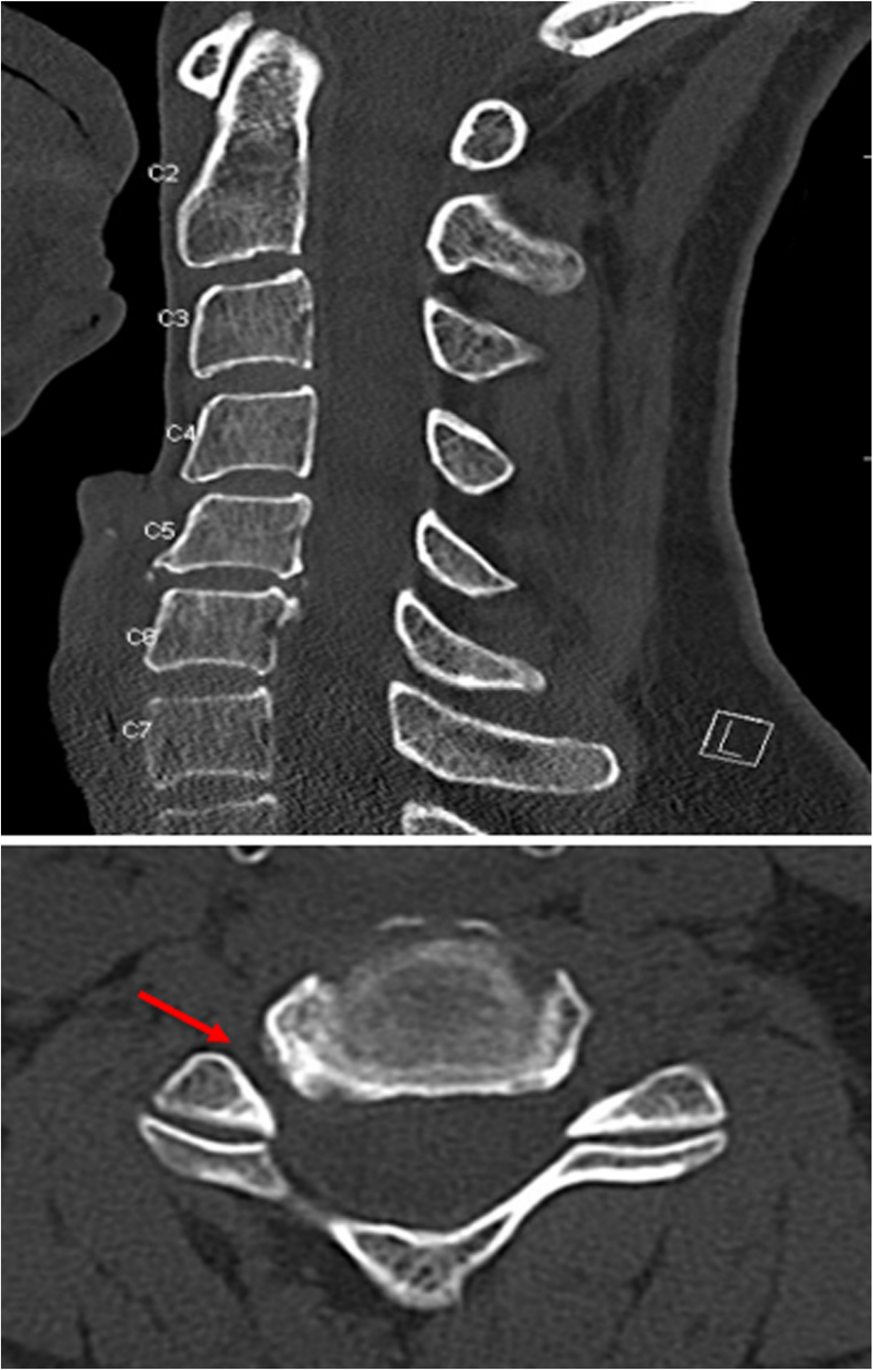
Pre-Operative CT assessment of patient after months-long and progressive C6 radicular symptoms. Moderate cervical spondylosis most pronounced at C4–5 and C5–6. At C5–6, there is considerable right neural foraminal narrowing (red arrow), mild left neural foraminal narrowing and mild spinal canal narrowing
